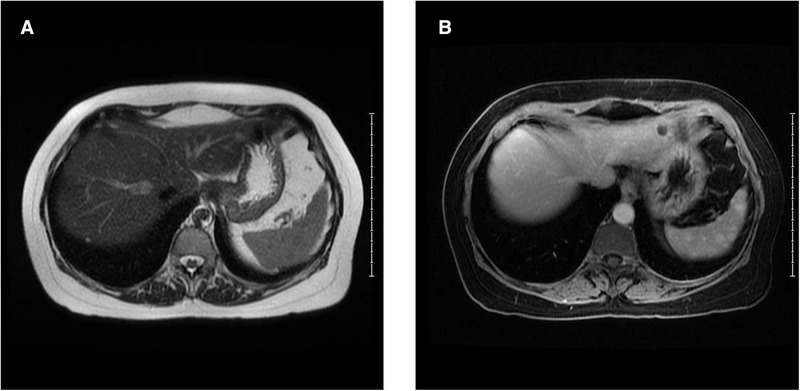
MRI of the abdomen T2 images showed splenic lesions as subtle hypo‐intensity (A). Focal splenic lesions became conspicuous on delayed post‐contrast images with enhancement (B) compared to the background parenchyma
